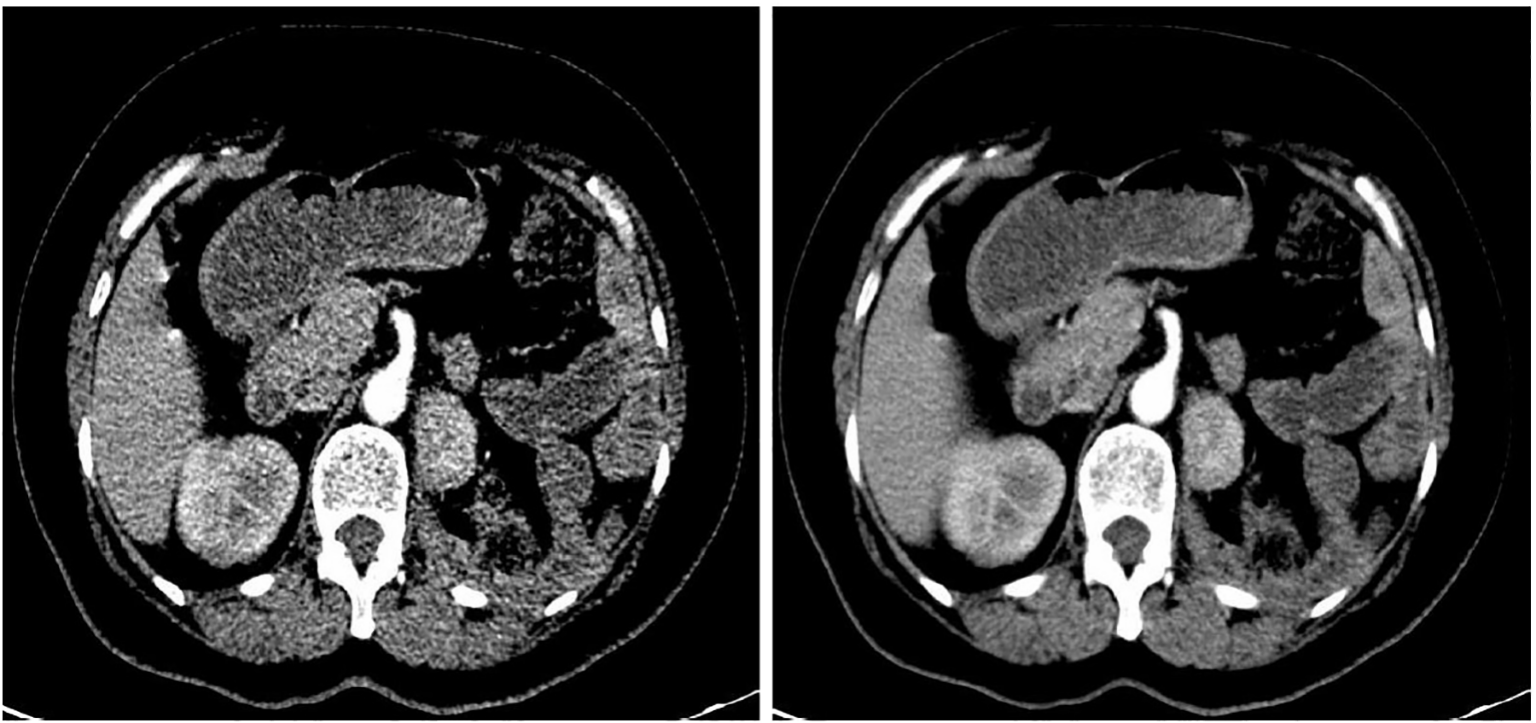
Contrast-enhanced CT revealed a tumor in the left renal fossa.
